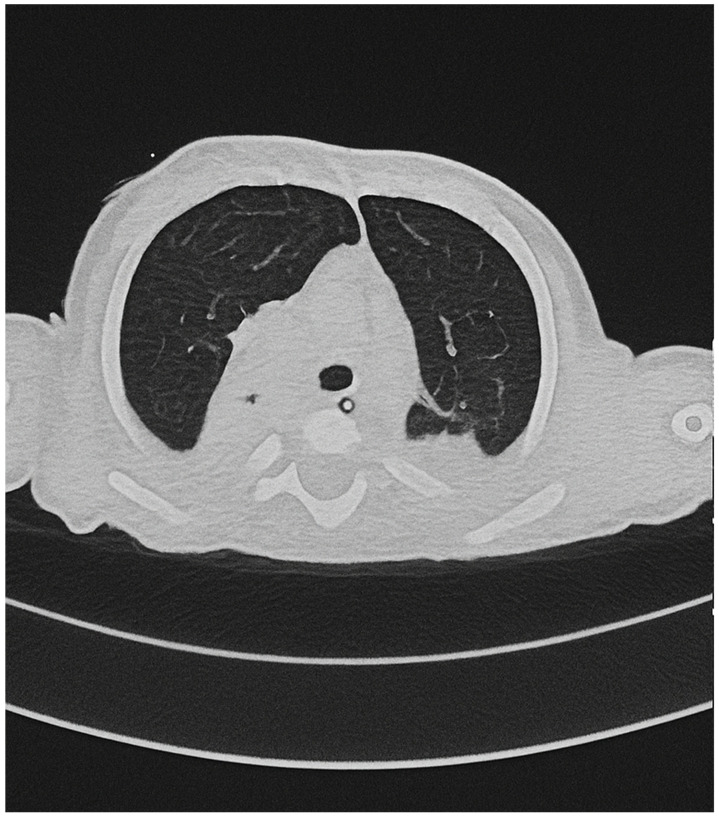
Contrast-enhanced CT angiography. The images demonstrate a right lower lobe lesion with systemic arterial supply from the celiac trunk, consistent with pulmonary pseudosequestration.
